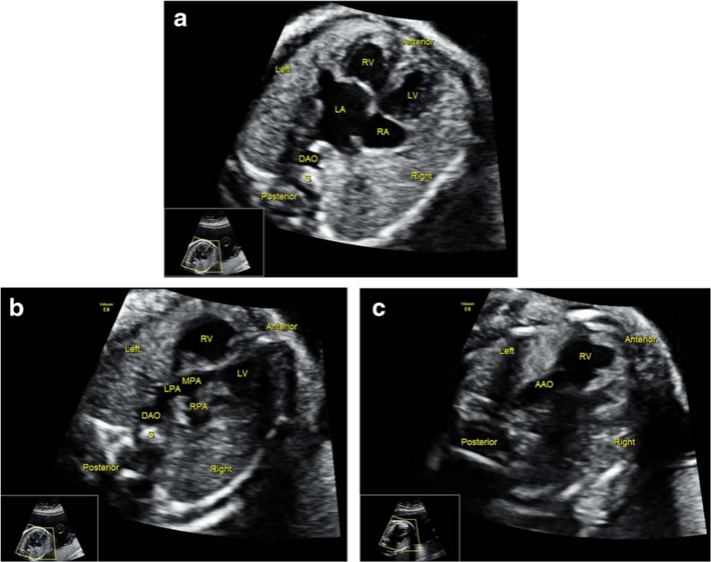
Sonographic images of a 22-gestational-week fetus diagnosed with ccTGA. The 4CV (a) showed four symmetric chambers that were considered to be normal by sonographers under Protocol A. However, it was confirmed as atrioventricular discordance by carefully visualizing the position of the atrioventricular valves that insert into the septum. In addition, the *left* (b) and *right* (c) outflow tract views substantiate that MPA was connected to morphological LV, while AAO connected to morphological RV. Sequential segmental analysis allowed sonographers under Protocol B to detect this anomaly. 4CV, four-chamber view; AAO, ascending aorta; ccTGA, congenitally corrected transposition of the great arteries; DAO, descending aorta; LA, left atrium; LPA, left pulmonary artery; LV, left ventricle; MPA, main pulmonary artery; RA, right atrium; RPA, right pulmonary artery; RV, right ventricle; S, spine
